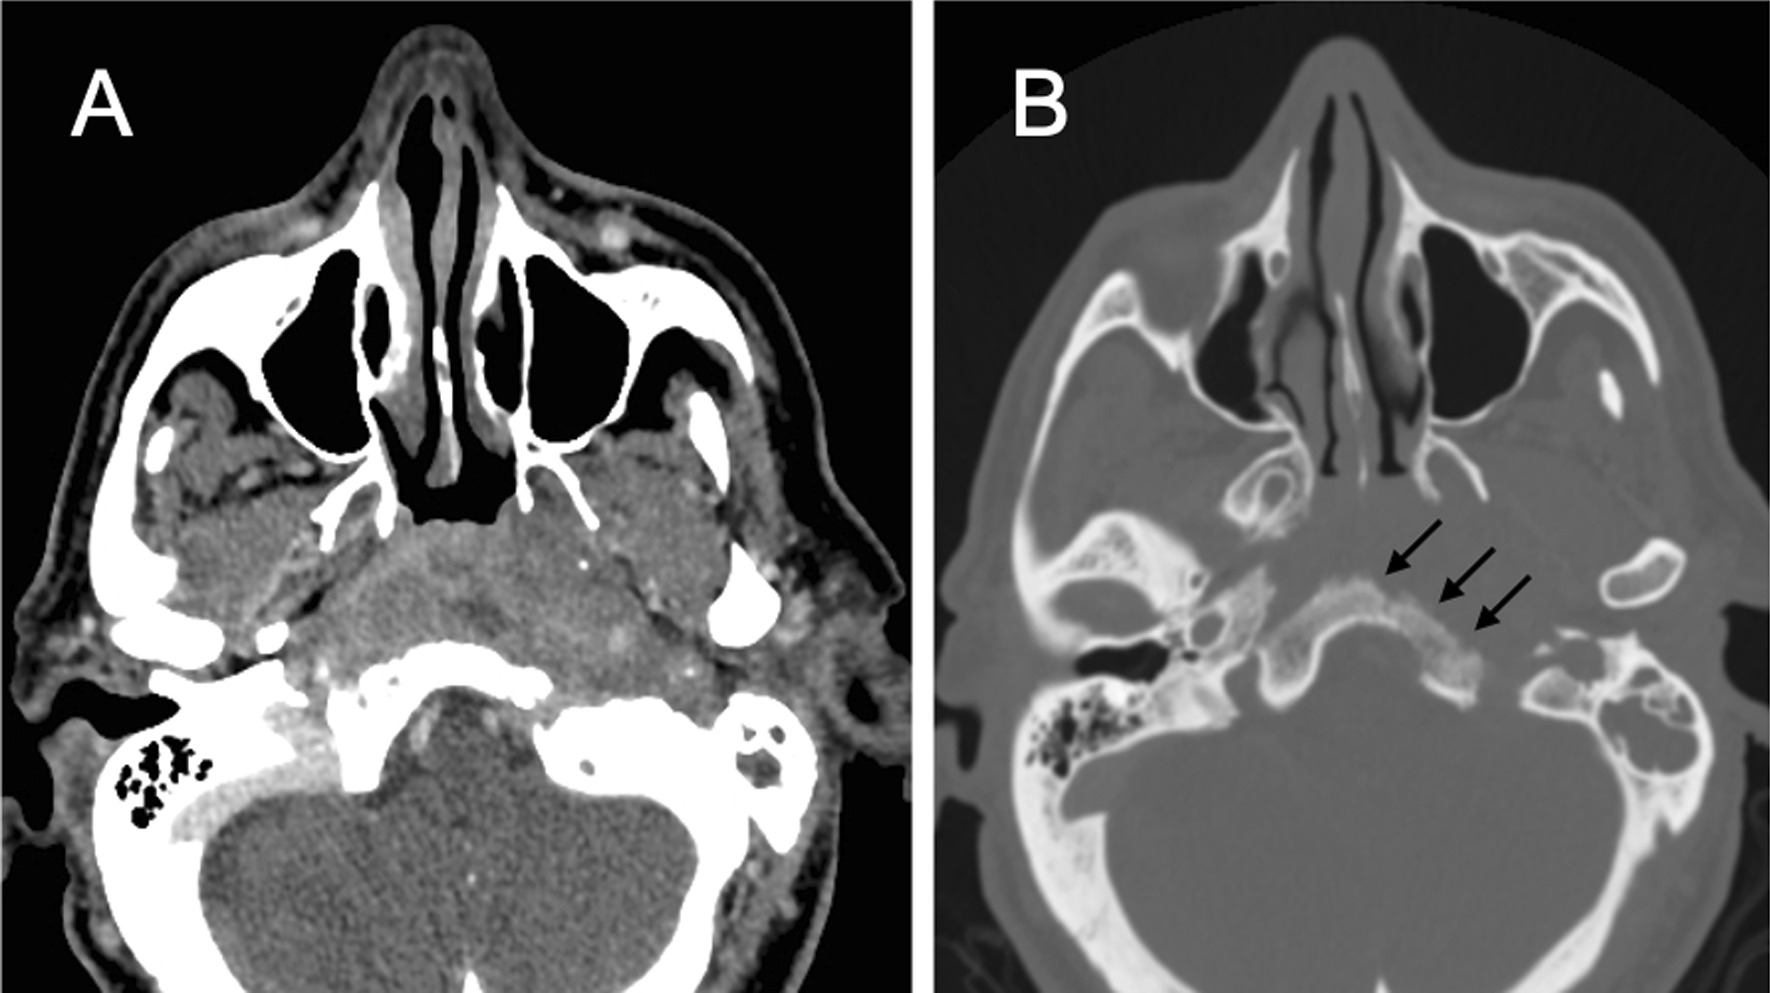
CT scan. A huge ill-defined soft tissue mass in the nasopharynx and perivascular space was seen in the midline on postcontrast CT scan. Heterogenous enhancement of the lesion (a) and associated bone erosion (b, *black arrows*) were observed. Occupation of mastoid cells on the left side were also noted
